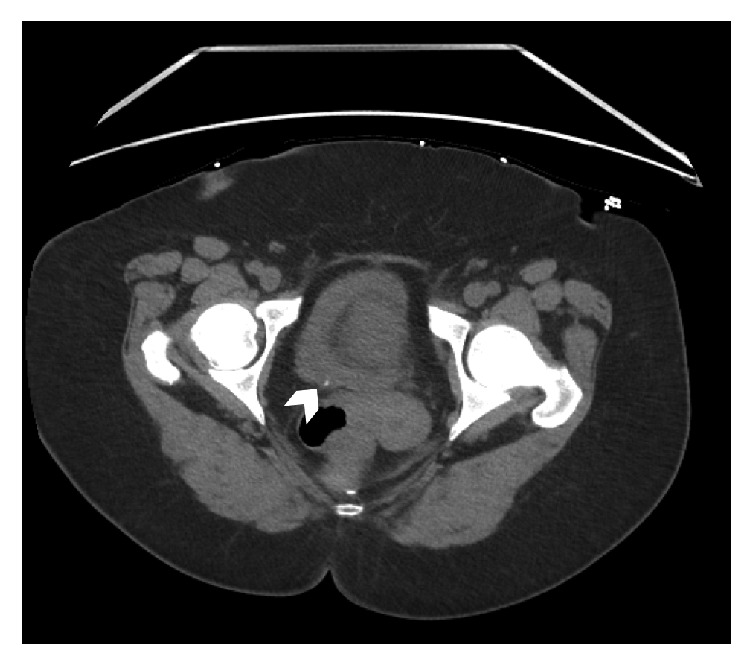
Axial CT scan of the abdomen and pelvis without contrast revealed an atrophic left kidney and a 4 mm stone (arrowhead) in the right ureterovesical junction causing mild hydroureteronephrosis with findings suggestive of pyelonephritis.
